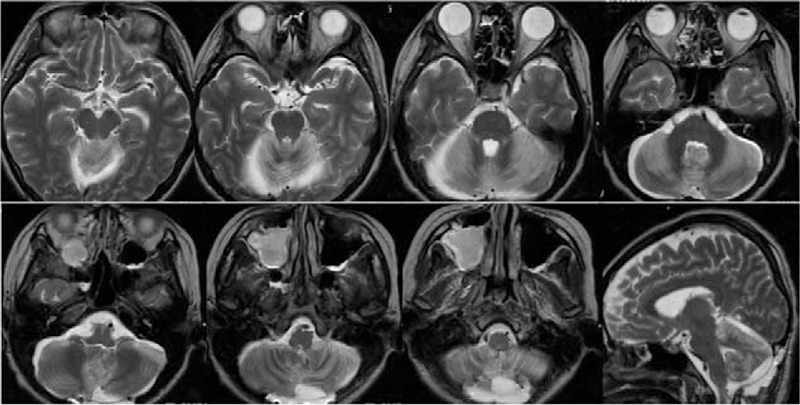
A contrast-enhanced abdominal CT suggested superior mesentery artery (SMA) syndrome. The axial view (A) demonstrated a distance between SMA and abdominal aorta was 6 mm and the proximal part duodenum third portion (asterisk) was dilated while the distal part was compressed. In sagittal view (B), the aorta-SMA angle was 15°. In coronal view, a bloated stomach occupied left upper abdominal quadrant and an obstructed duodenum can be seen (asterisk). CT = computed tomography.
